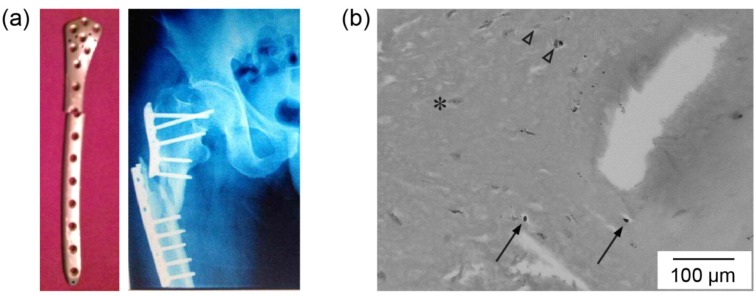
(a) The Ti exterior plate and the X-ray film of the patient; (b) the cross-section of the tissue collected from a hole of a locking screw (not on the broken site). Arrows point at the debris particles after encapsulation. Triangles point at the particles with surrounding inflammatory cells. Fibroblast is marked by an asterisk.
